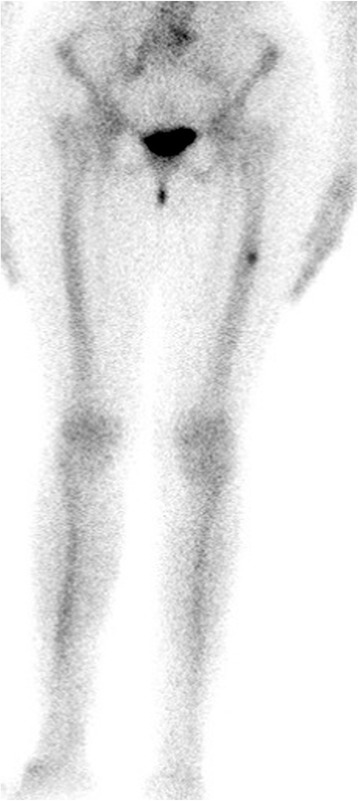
Bone scintigraphy of a case of incomplete AFF
